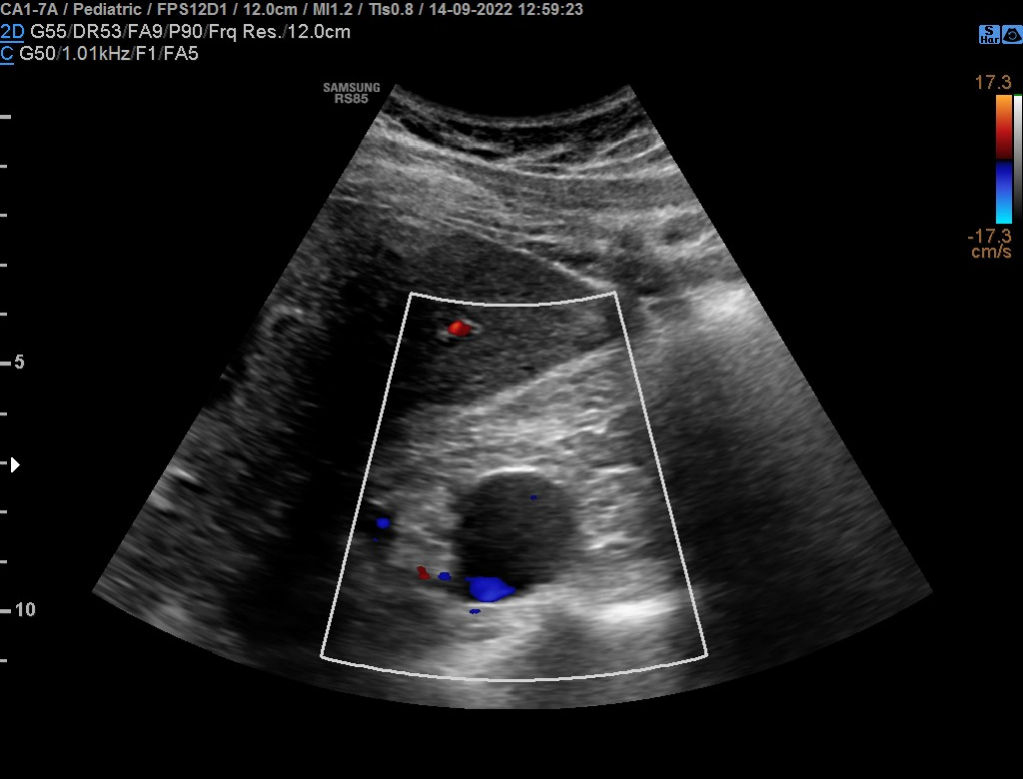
Abdominal ultrasonography shows multicellular cyst in the hilar region of the right kidney.
